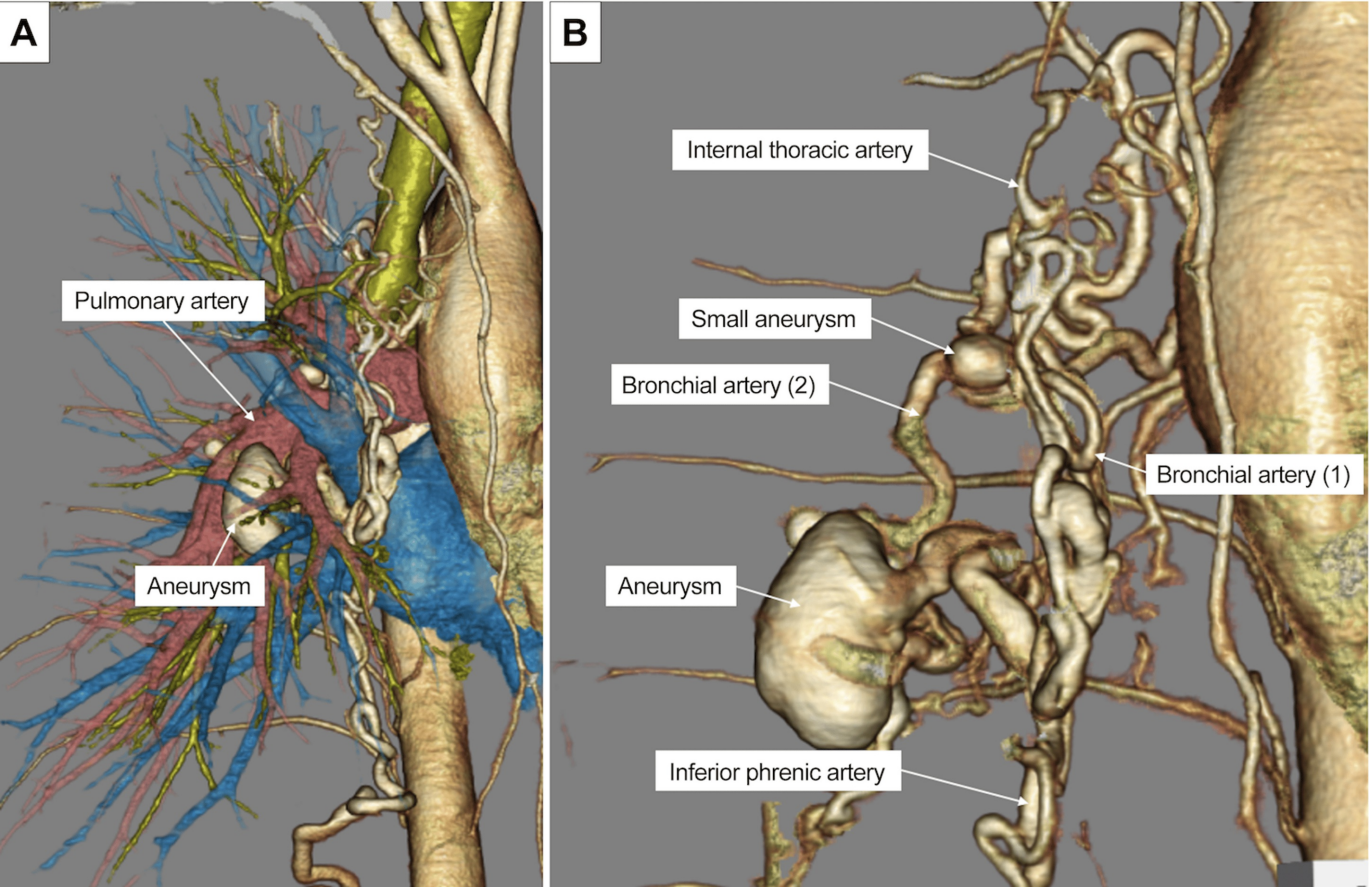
Three-dimensional computed tomography (CT) of the multiple systemic arteries-to-pulmonary artery fistula (A) Several abnormal systemic arteries flow into the right lower pulmonary artery after passing through a 3-cm aneurysm. (B) The right inferior phrenic, right internal thoracic, and two right bronchial arteries, along with a small aneurysm, are visualized, flowing into the aneurysm.
